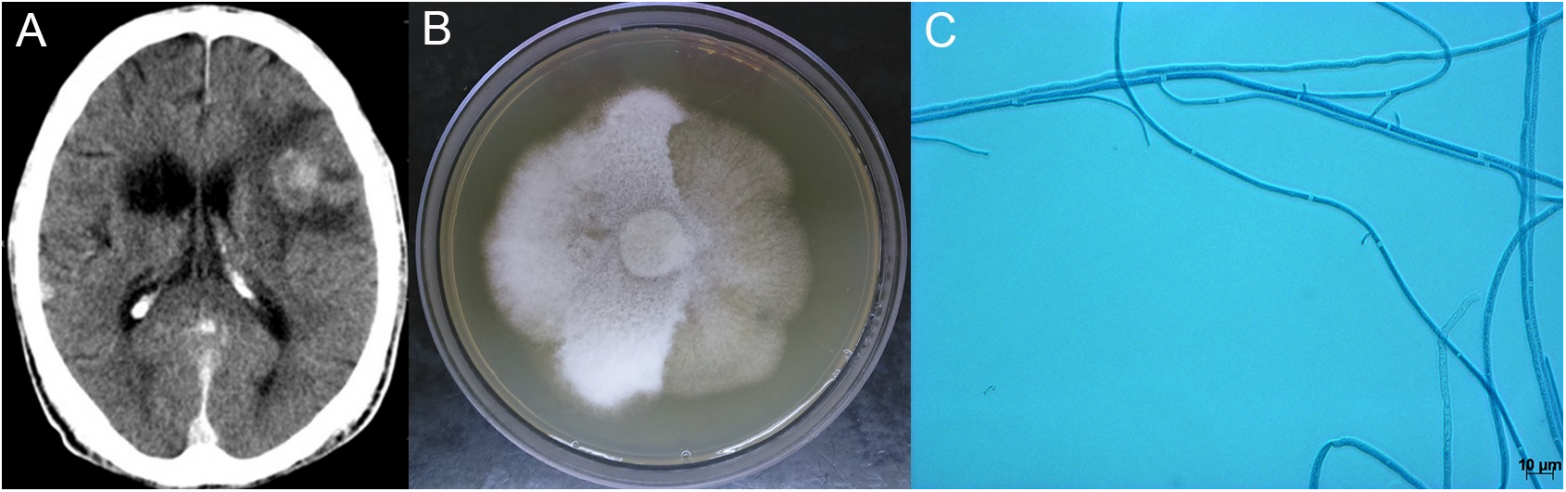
Schizophyllum commune* infection in an HIV-infected patient. (A) Brain CT scan showing contrast-enhancing lesions in both cerebral hemispheres associated with mass effect and a hypodense lesion in the right caudate nucleus suggestive of encephalomalacia from previous lesions. (B)
